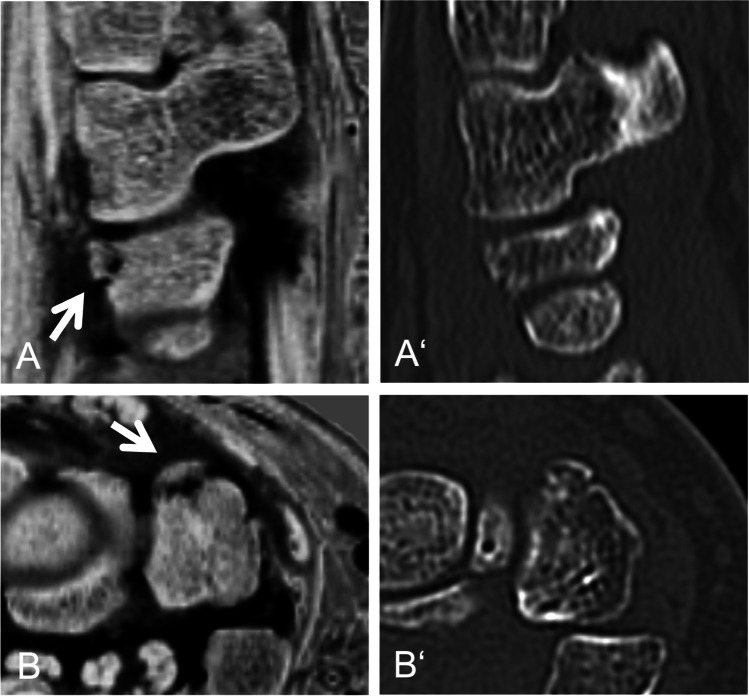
Forty-year-old patient with an acute traumatic avulsion fracture of the triquetrum (arrow). Multiplanar reconstruction in sagittal (A, A‘) and axial plane (B, B‘) based on an inverted 3D T1GRE sequence (A, B) and a CT (A‘, B‘). Note, the clearly depicted fracture line compared to the conventional CT in the axially reformated image (B‘)
